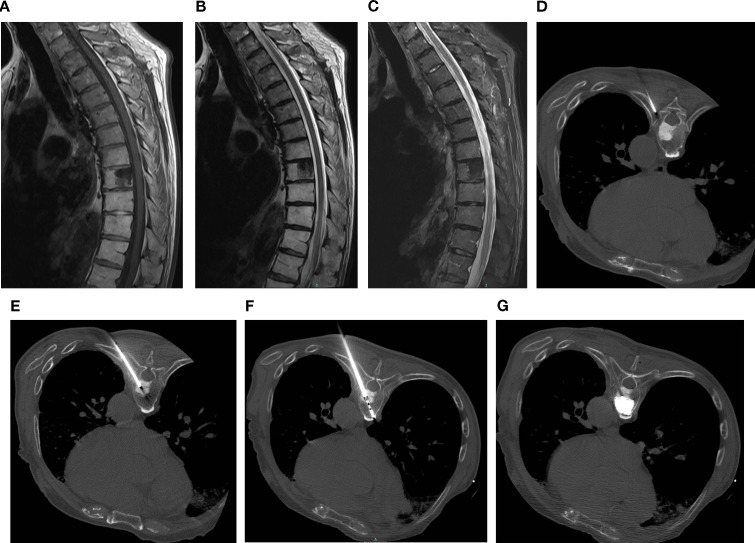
Fine needle-assisted puncture positioning was employed during computed tomography-guided percutaneous vertebroplasty combined with microwave ablation in a patient with lung cancer metastatic to the sixth thoracic vertebral body. (A–C) MRI scans showed a tumor mass in the sixth thoracic vertebral body. (D) A 20-gauge PTC fine needle was inserted near the tumor mass. (E) A bone puncture needle was gradually inserted into the tumor according to the mark of the fine needle. (F) CT scan showed that the microwave ablation needle was located in the tumor body. (G) bone cement (3 mL) was injected after ablation (20 W, 2 minutes).
